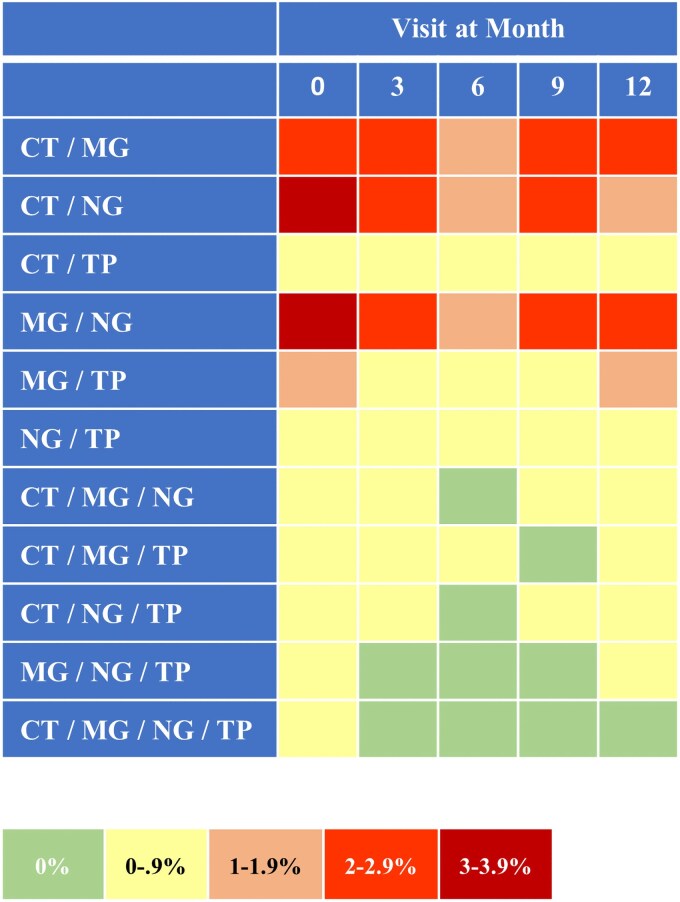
Prevalence of concurrent coinfections at the same study visit with *Chlamydia trachomatis* (CT), *Mycoplasma genitalium* (MG), *Neisseria gonorrhoeae* (NG), and *Treponema pallidum* (TP) by study visit. Absolute numbers of participants by visit: month 0, n = 1017; month 3, n = 1009; month 6, n = 1000; month 9, n = 985; month 12, n = 969.
